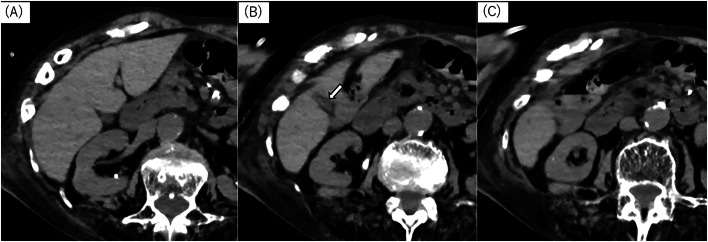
Follow-up noncontrast CT, axial image. Follow-up noncontrast CT confirmed that not only the abscess but also the gallbladder disappeared without biliary dilatation or bile leakage (Fig. 3, arrow).
